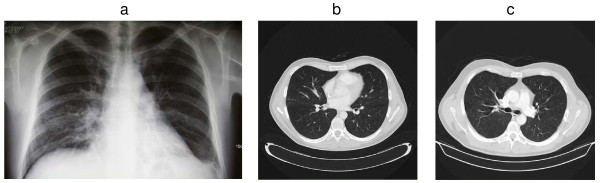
X-ray and computed tomography (CT) scans of patient with severe *Plasmodium vivax *malaria. Interstitial bilateral infiltrates compatible with acute lung injury in X-ray (Panel A). Infiltrates in right midzone in CT scan (panel B). Normal CT scan two months after acute episode (panel C).
